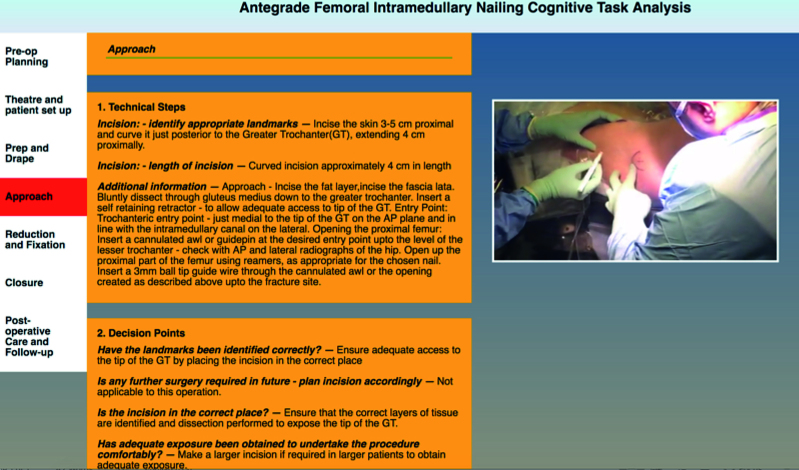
Snapshot of the IFINCTA tool showing the written information of the CTA on the left and the multimedia video clips and audio recordings on the right for the “approach” phase of the procedure.
